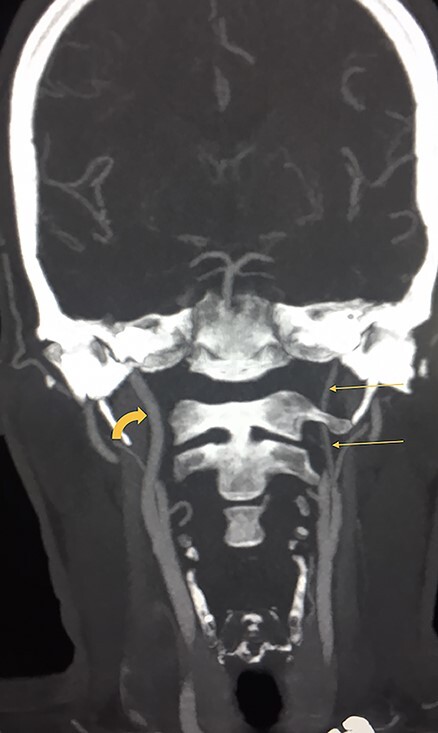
CT angiogram coronal maximum intensity projection (MIP, 0.625 mm slice thickness) reconstruction image shows a long-tapered stenosis in the left ICA, originating beyond the carotid bulb (straight arrows). This is in keeping with a dissection. Normal ICA on the right (curved arrow).
